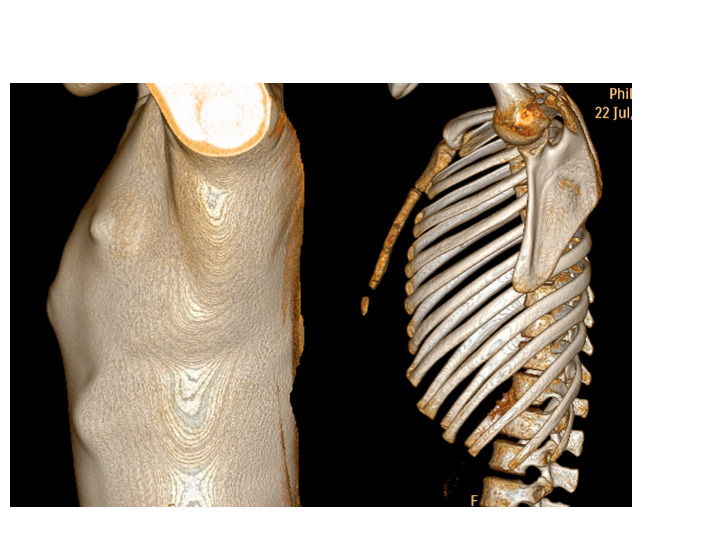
Chest computed tomography scan with 3D reconstruction of patient with pectus carinatum.
